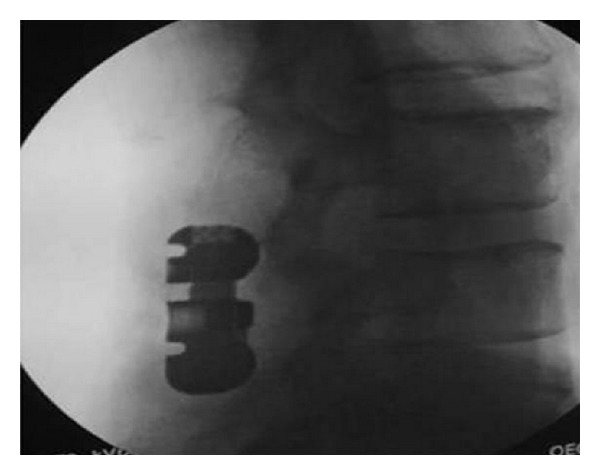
Intraoperative lateral X-ray of the BridgePoint System with the telescoping plates that fixate and compress spinous processes restoring sagittal alignment.
